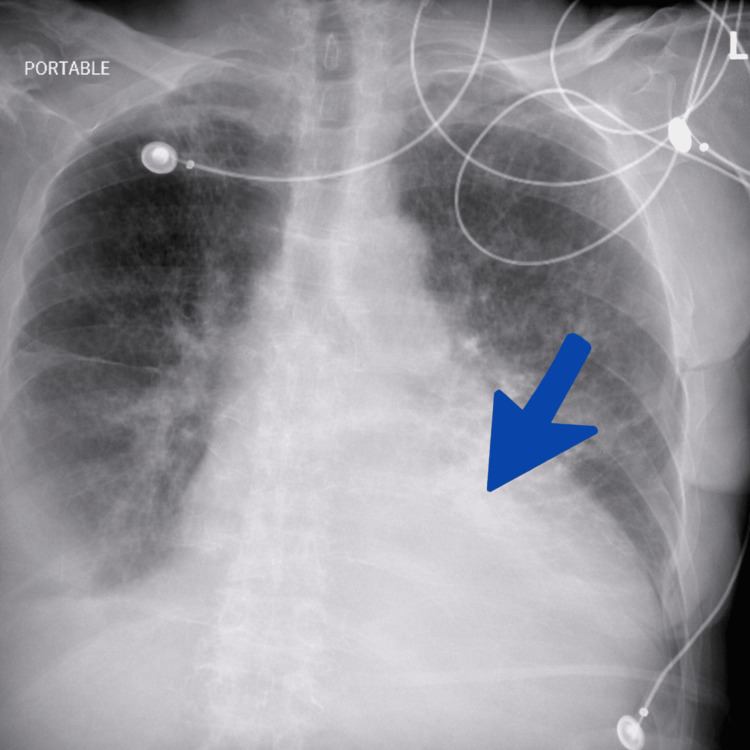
Portable chest radiograph demonstrating cardiomegaly and pulmonary congestion. A portable anteroposterior chest radiograph shows cardiomegaly with bilateral bibasilar opacities, consistent with pulmonary congestion in advanced heart failure.
